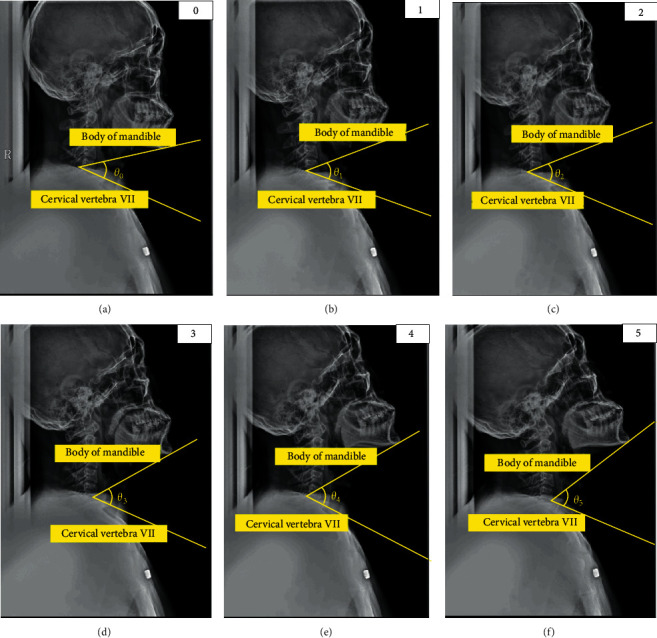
Five oropharynx area X-ray images obtained at random angles: (a) origin point (*θ*_0_), (b) 5.03° expansion (*θ*_1_) from *θ*_0_, (c) 5.01° expansion (*θ*_2_) from *θ*_1_,(d) 4.95° expansion (*θ*_3_) from *θ*_2_, (e) 4.99° expansion (*θ*_4_) from *θ*_3_, and (f) 5.02° expansion (*θ*_5_) from *θ*_4_.
